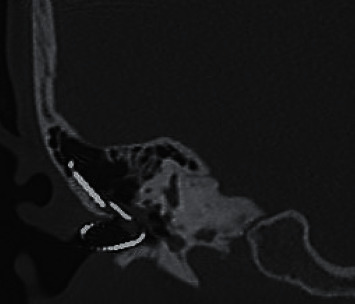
Coronal CT scan of the right temporal bone confirming explantation had taken place with the electrode array coiled up in the external auditory canal.
